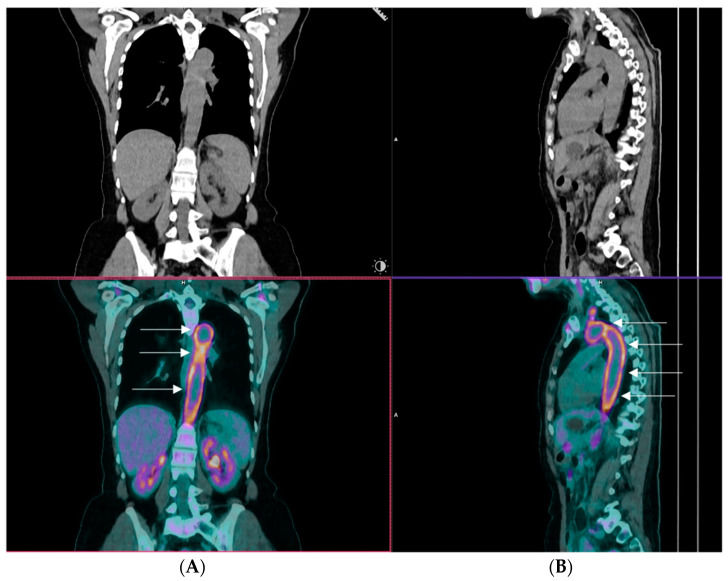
A representative image of an 18F-fluorodeoxyglucose positron emission tomography/computed tomography (PET/CT) of a patient with giant cell arteritis and extracranial large vessel involvement. The image displays coronal (Panel (A)) and sagittal views (Panel (B)), highlighting robust tracer uptake within the arch of the aorta, which is shown by arrows (kindly provided by our collaborator Dr CD Anagnostopoulos (BRFAA)).
